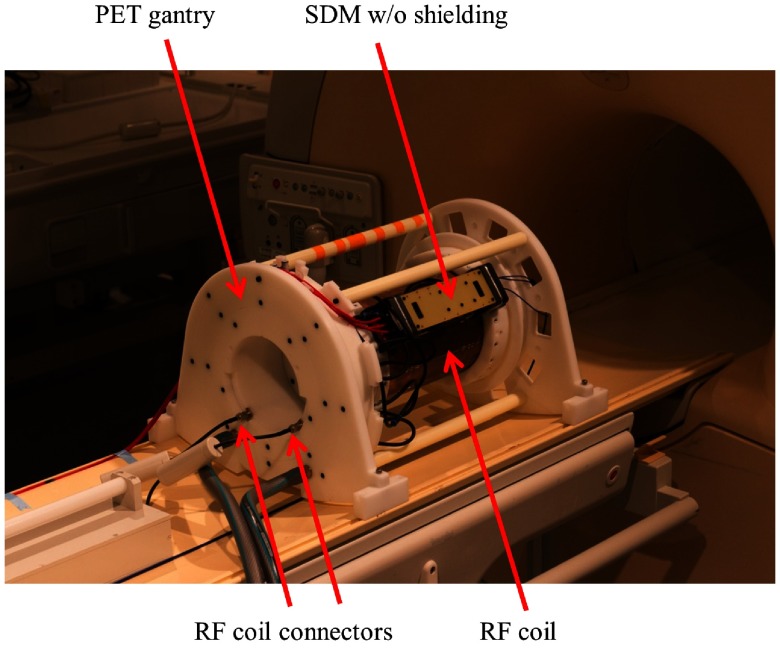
A single SDM without carbon fibre shielding mounted on a PET gantry to make MRI noise measurements. The RF coil was connected via coil electronics to the MRI RF subsystem.
